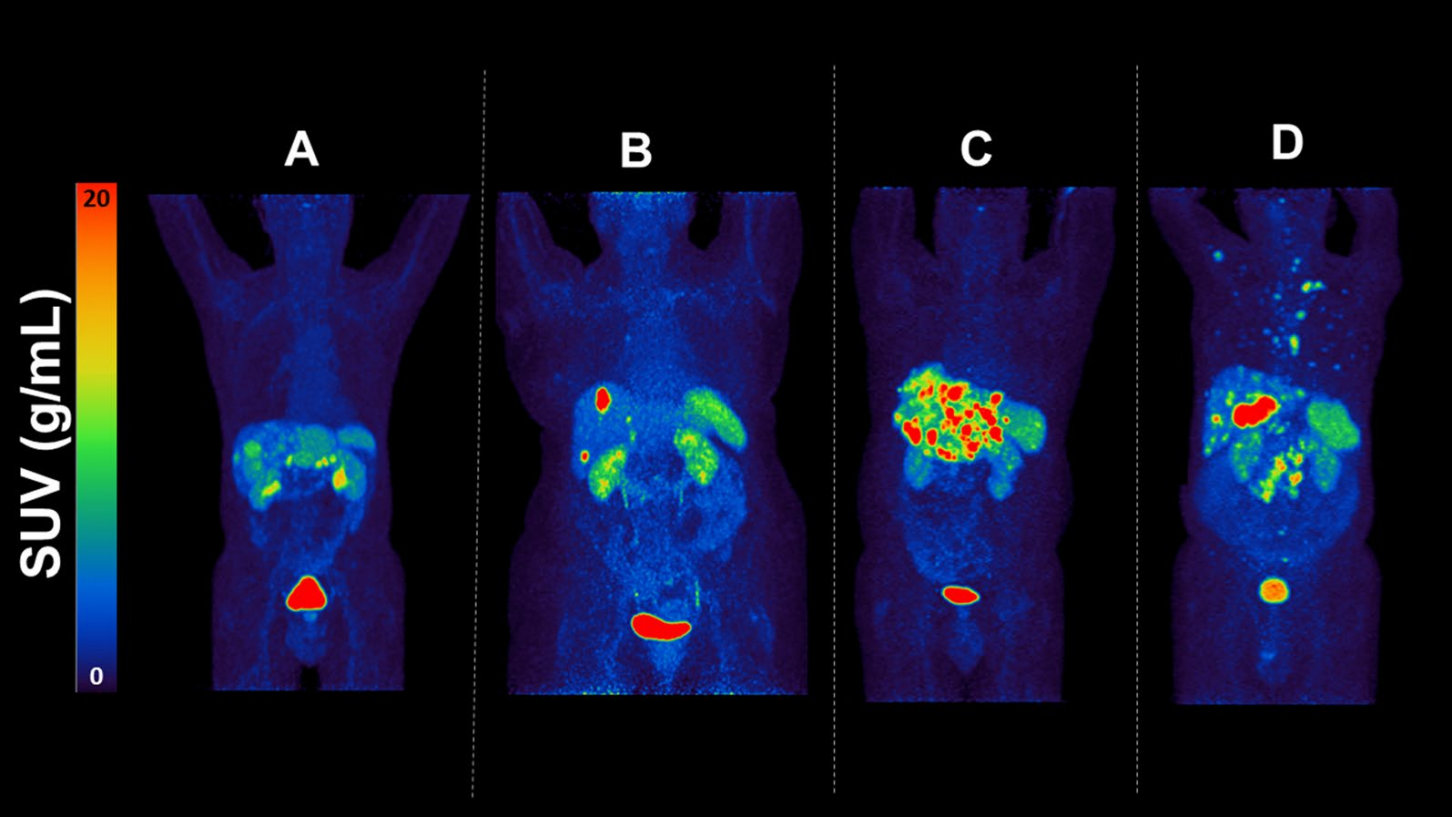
Maximum intensity projections of patient 1–4 (A–D) at baseline whole-body ^68^Ga-DOTATOC showing SUV values, where red color represents SUV of 20 and blue SUV of 0
